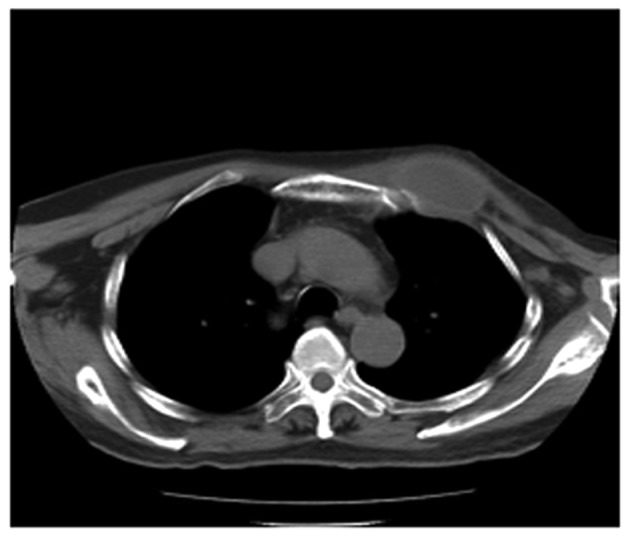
Chest wall tuberculosis prior to transdermal ultrasound treatment.
